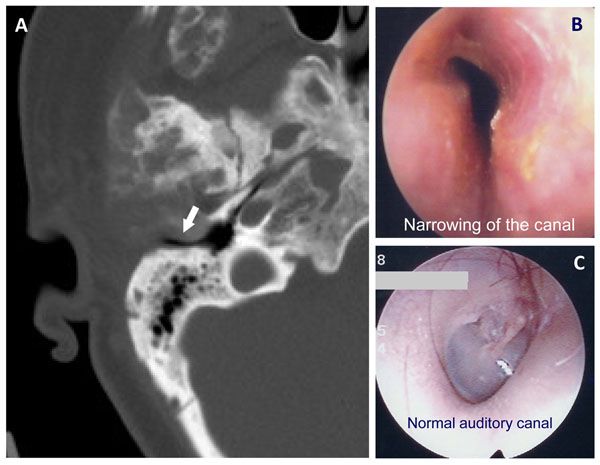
Narrowing of the external auditory canal due to fibrous dysplasia (FD). A) A CT image of a coronal slice through the temporal bone shows a narrowed external auditory canal (arrow) B) Narrowing of the canal is shown and can be compared to a normal canal in (C). The arrow on the CT image (A) demonstrates narrowing of the canal. This has resulted in hearing loss. The clinical images on the right compare a canal narrowed by FD to a normal external auditory canal.
